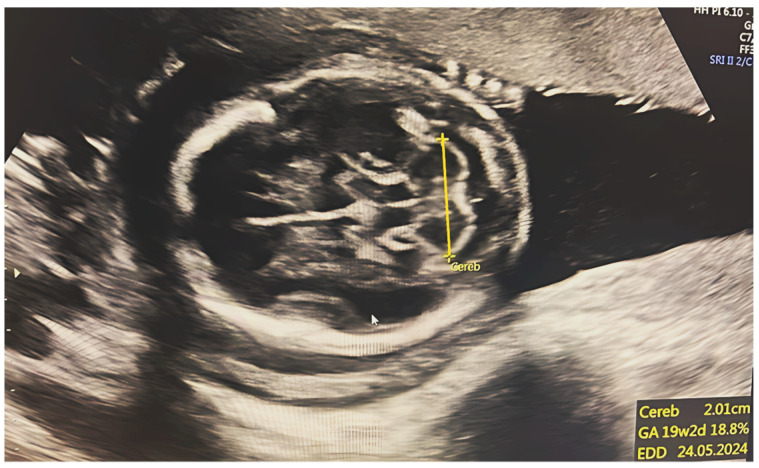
Selected ultrasonography image of transverse cerebellar dimension measurement to estimate fetal gestational age, which was found to be 19 weeks ± 2 days.
